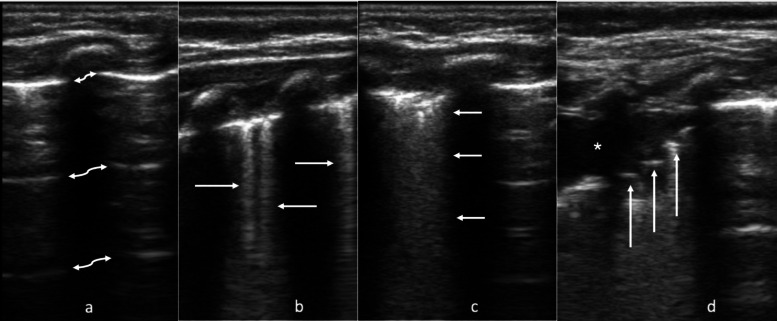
Four lung ultrasound findings. Example of the four lung ultrasound findings and associated scores, as previously reported^12^. (a) shows A lines (white arrows) with < 3 B lines (no B lines shown in this image), scores 0, (b) shows ≥ 3 B lines per lung segment (3 B lines shown in this image by white arrows), scores 1, (c) shows consolidated B lines, or “white out,” (white arrows) scores 2, (d) shows subpleural consolidation (shown with white *) with consolidated B lines, scores 3. In this final image, white arrows indicate air bronchograms, a common feature in subpleural consolidations
